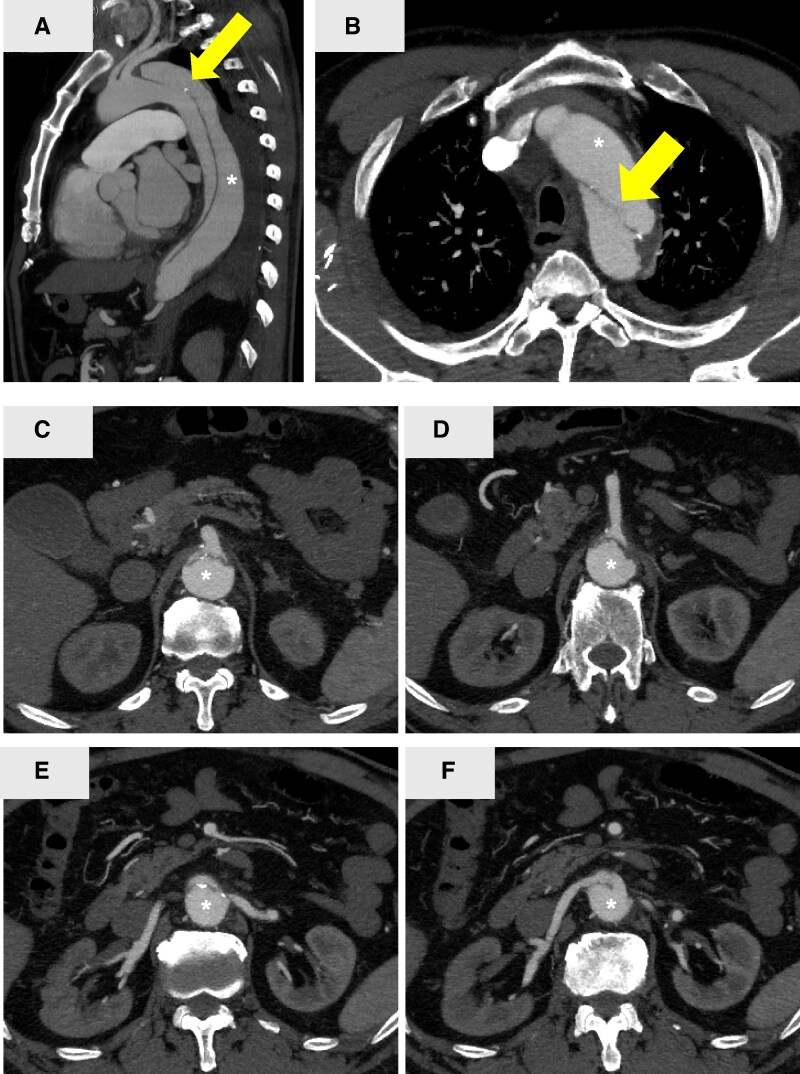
Preoperative enhanced computed tomography images of Stanford type B aortic dissection. An asterisk (*) indicates the false lumen. The primary entry tear is indicated by a yellow arrow in the sagittal (*A*) and axial (*B*) images of the distal aortic arch. The celiac artery (*C*), superior mesenteric artery (*D*), left renal artery (*E*), and right renal artery (*F*) branch from the true lumen. The density at the kidney in the early phase is lower than that at the aorta, suggesting renal ischaemia.
